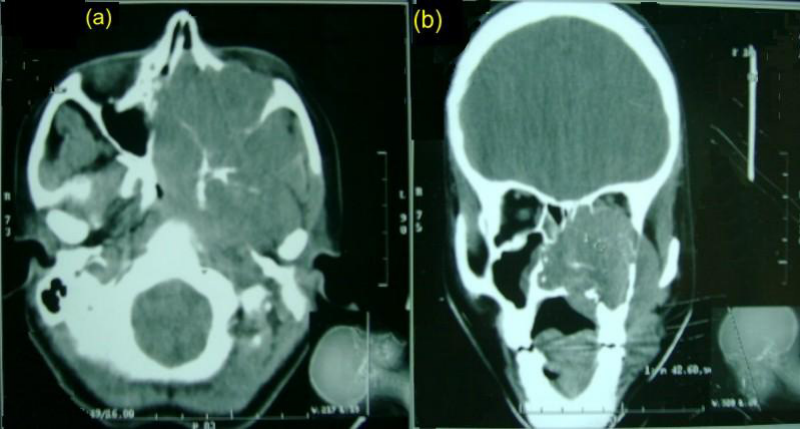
Pre-treatment axial and coronal paranasal sinuses and orbit contrast-enhanced CT-Scan (a, b). Showing bulky soft-tissue mass in left maxilla, bilateral ethmoid sinuses, nasal cavity, pterygopalatine fossa, nasopharynx with destruction of medial and lateral wall of orbit.
